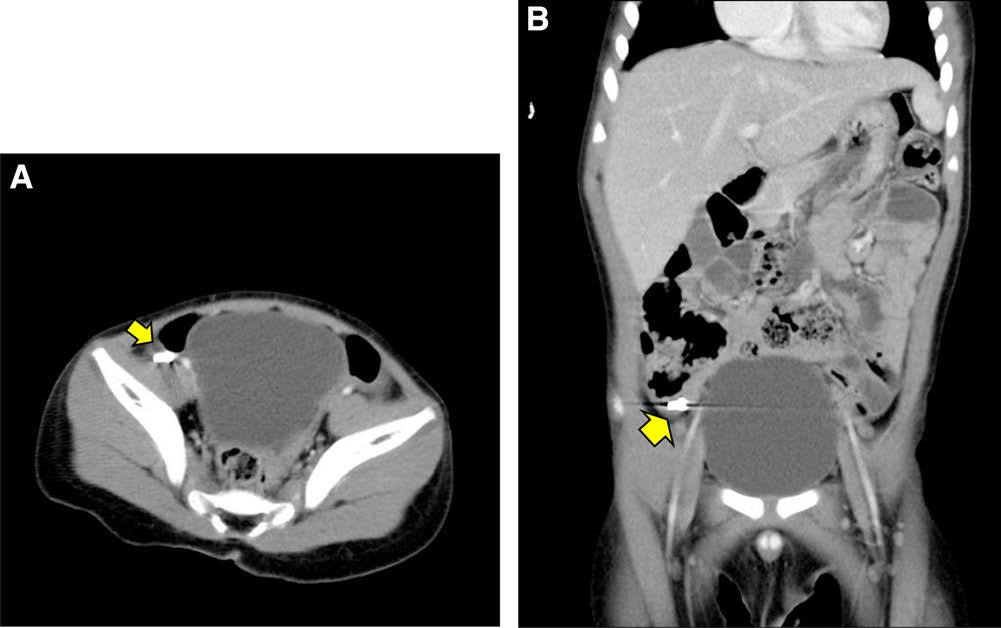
Screw shape metallic foreign body (arrow) over ileo-cecal valve with mild dilatation of small bowel under CT axial view (A) and coronal view (B).
